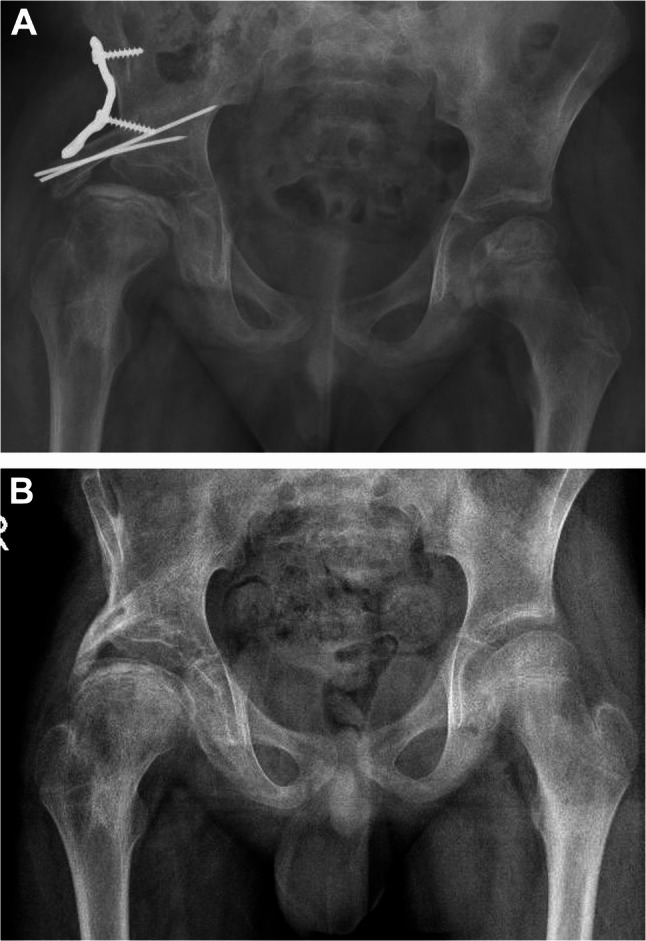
A A male patient diagnosed with a right hip ONFH lateral pillar grade C at the age of 9.5-y. Immediate post-operative radiograph following SA. B Radiograph after the removal of reconstruction plate and wires 18-m following SA, demonstrating a healed shelf graft and grade 3 Stulberg of the right hip
